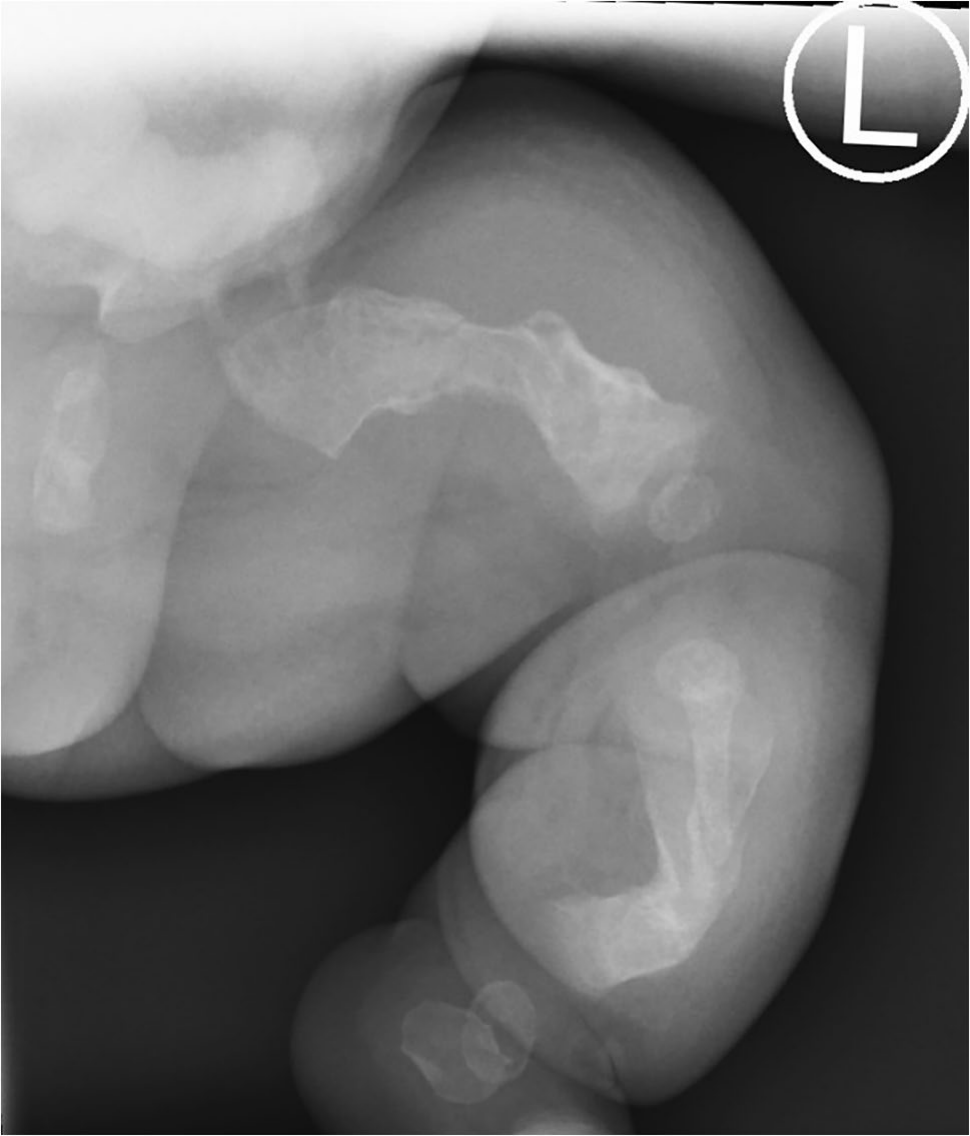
Plain radiograph of left lower limb of the same infant with severe osteogenesis imperfecta shown in Fig. 1. There are fractures as well as shortening, widening, and angulation of the femur, tibia, and fibula. These skeletal features are indicative of generalised profound material weakness and low bone mass
